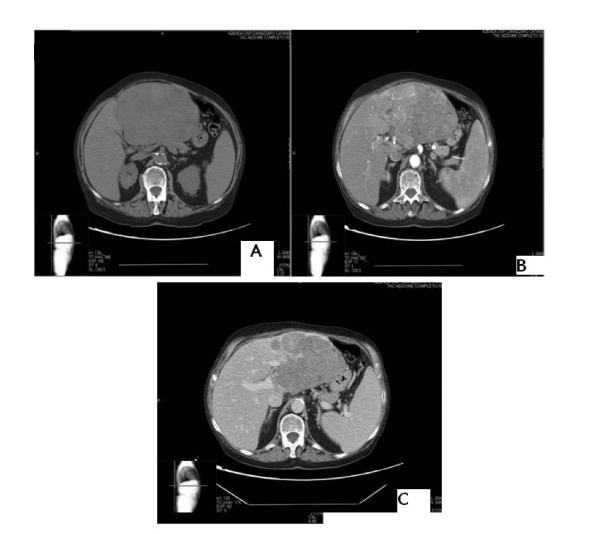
Computed tomography scan of the tumor in liver segments 2 and 3. (A) Pre-contrast phase; (B) Arterial phase; (C) Portal phase.
